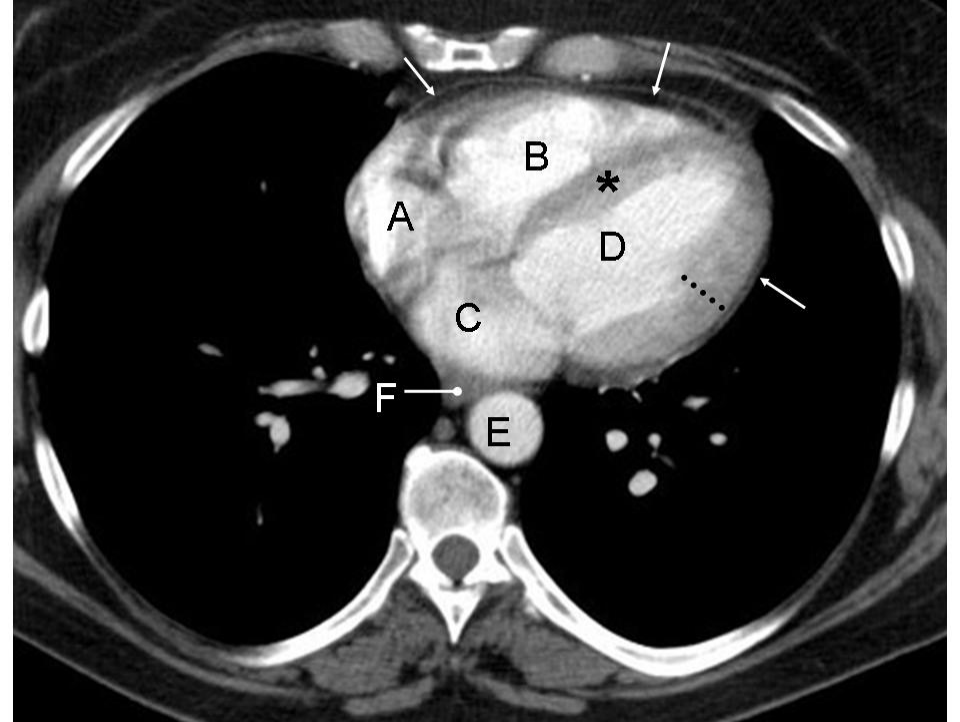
A contrast enhanced axial CT image of the chest of a 45 years old male is shown. Identify the labelled normal anatomical structures
